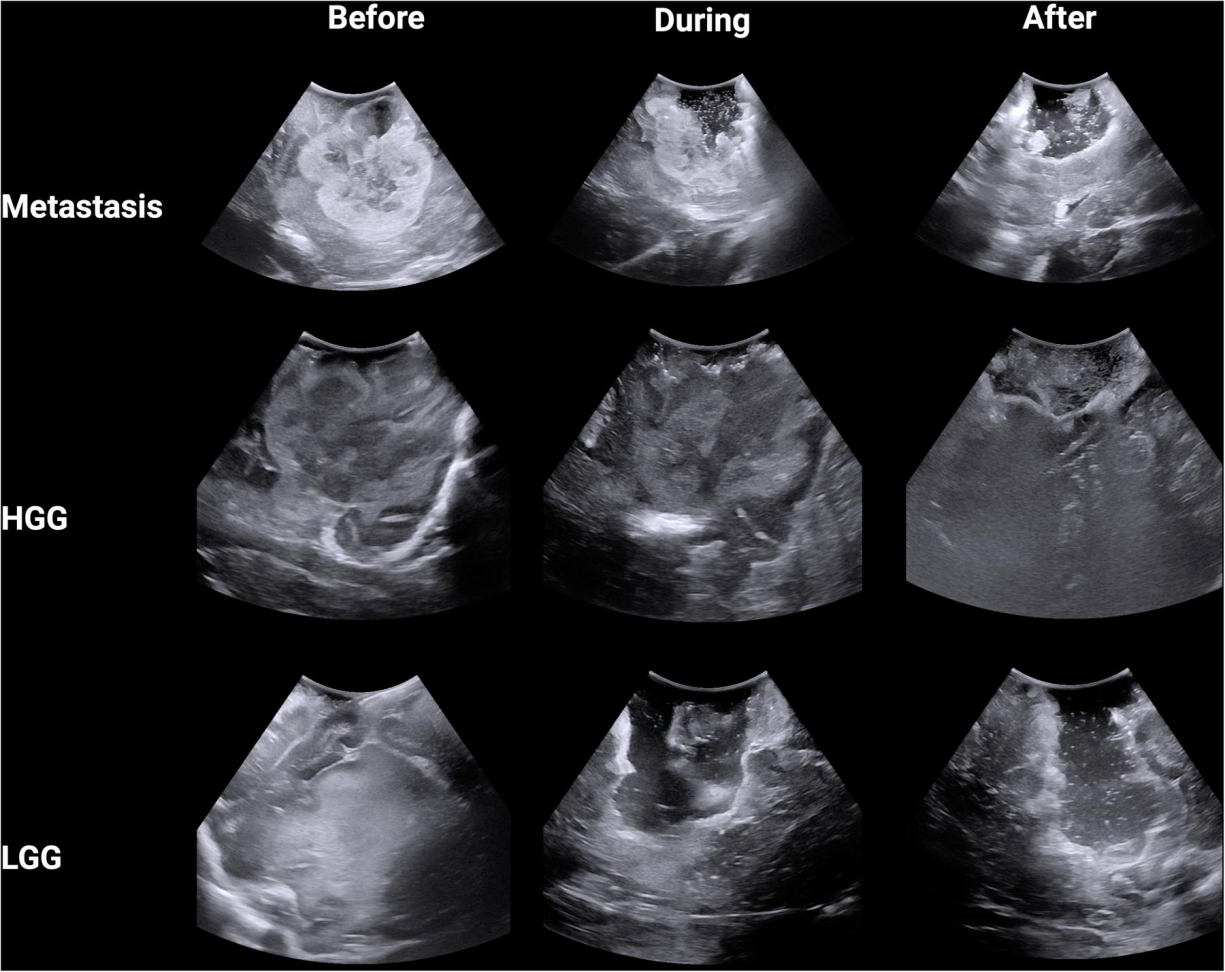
Intra-operative B-mode ultrasound images from three tumour types across three stages of surgery.
